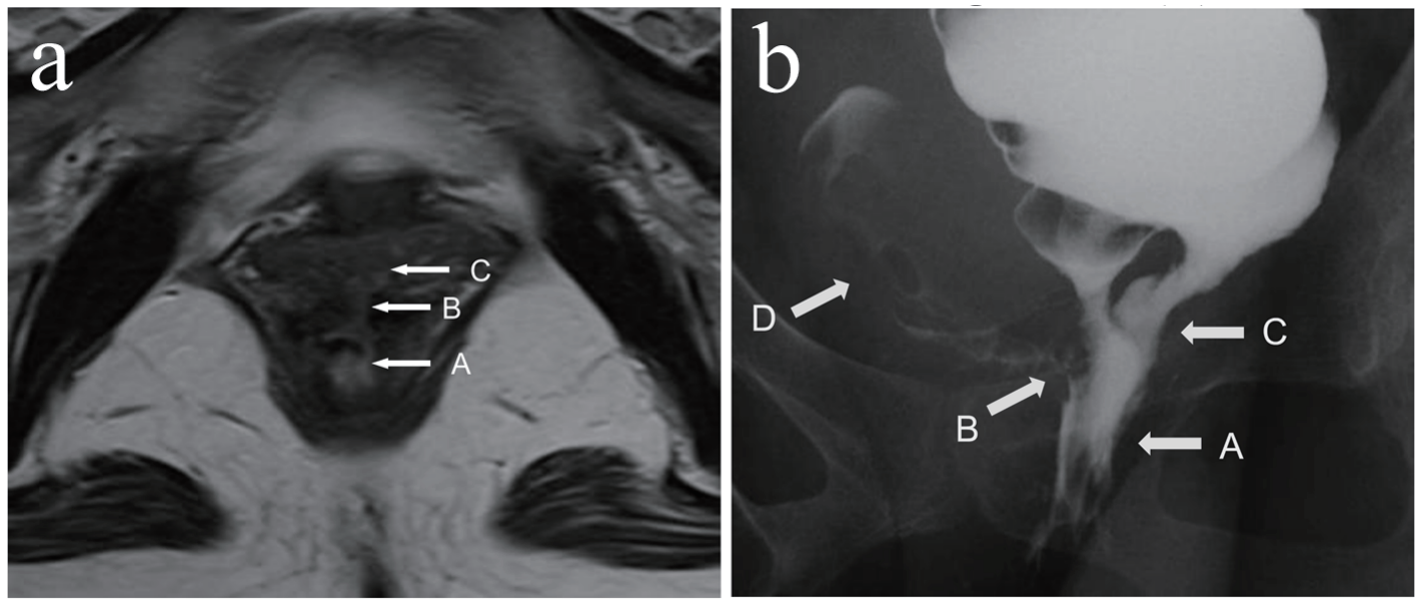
(a) MRI showed the rectovaginal fistula before administration of infliximab. (b) Barium-enema study showed the barium running into the vaginal cavity before administration of infliximab. White arrows pointed respectively A: rectum; B: rectovaginal fistula; C: J-pouch (atypical bridge); D: vagina cavity.
